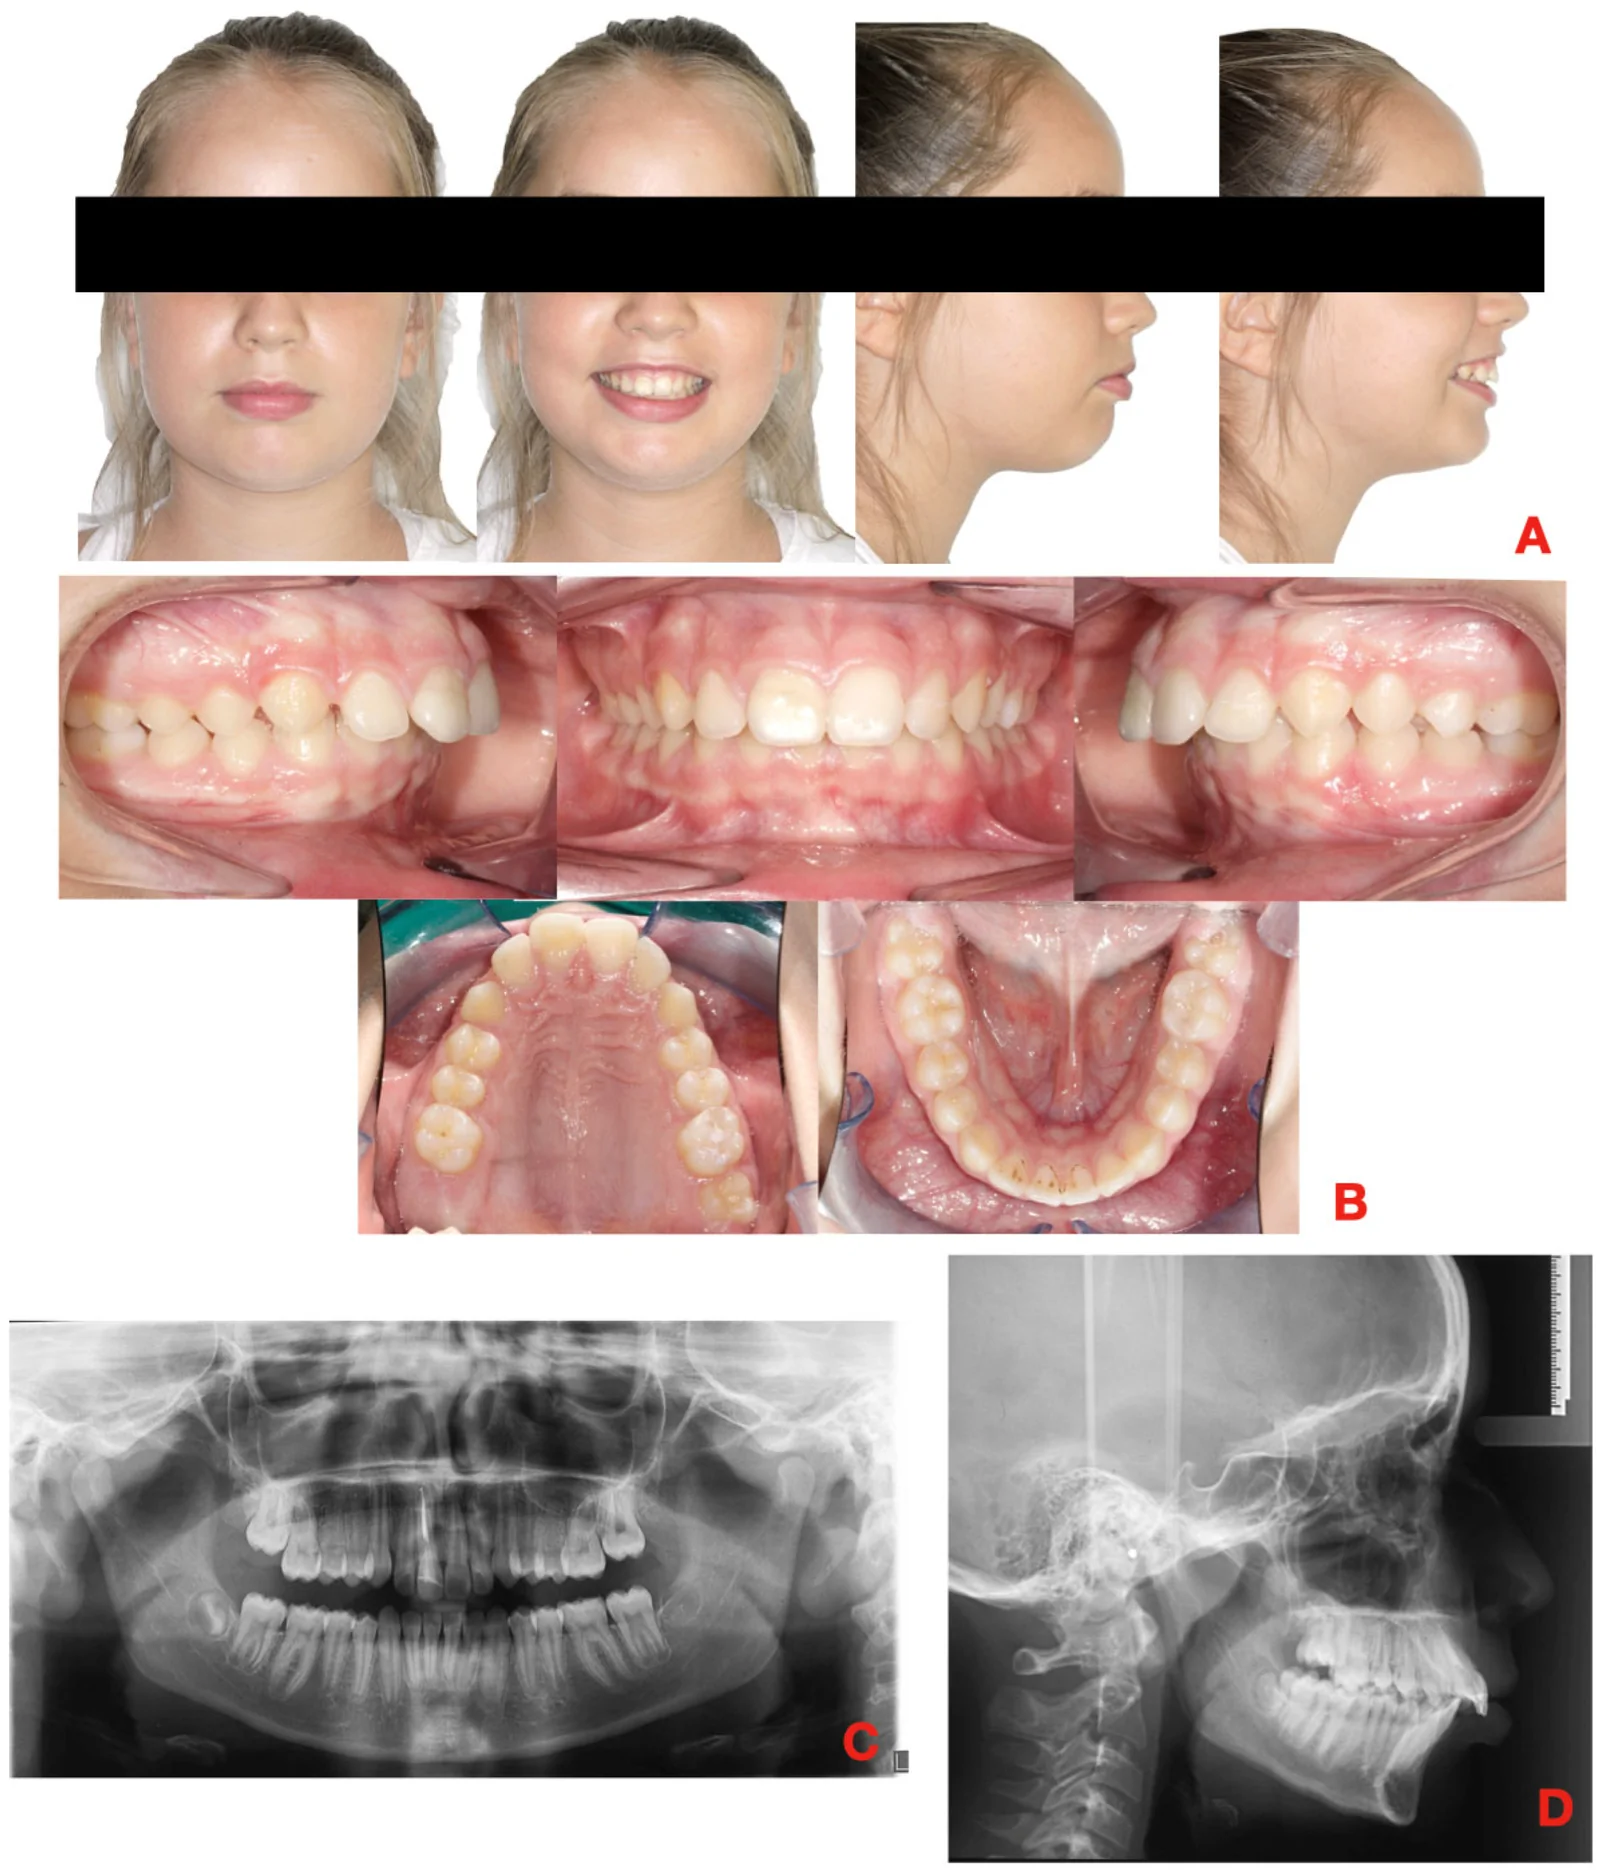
Initial records of the 11-year-old female patient at the beginning of treatment (T0). (A). Extra-oral photographs in frontal and lateral views. (B). Intra-oral photographs in frontal, lateral, and occlusal views. (C). Orthopantomography. (D). Lateral cephalogram radiograph.
